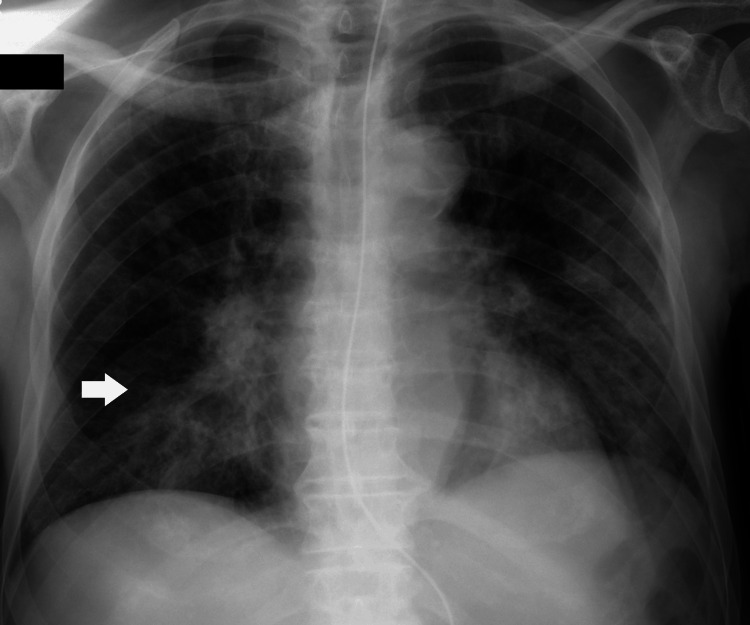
Chest radiograph showing a right lower lung zone consolidation, consistent with pneumonia (arrow).
